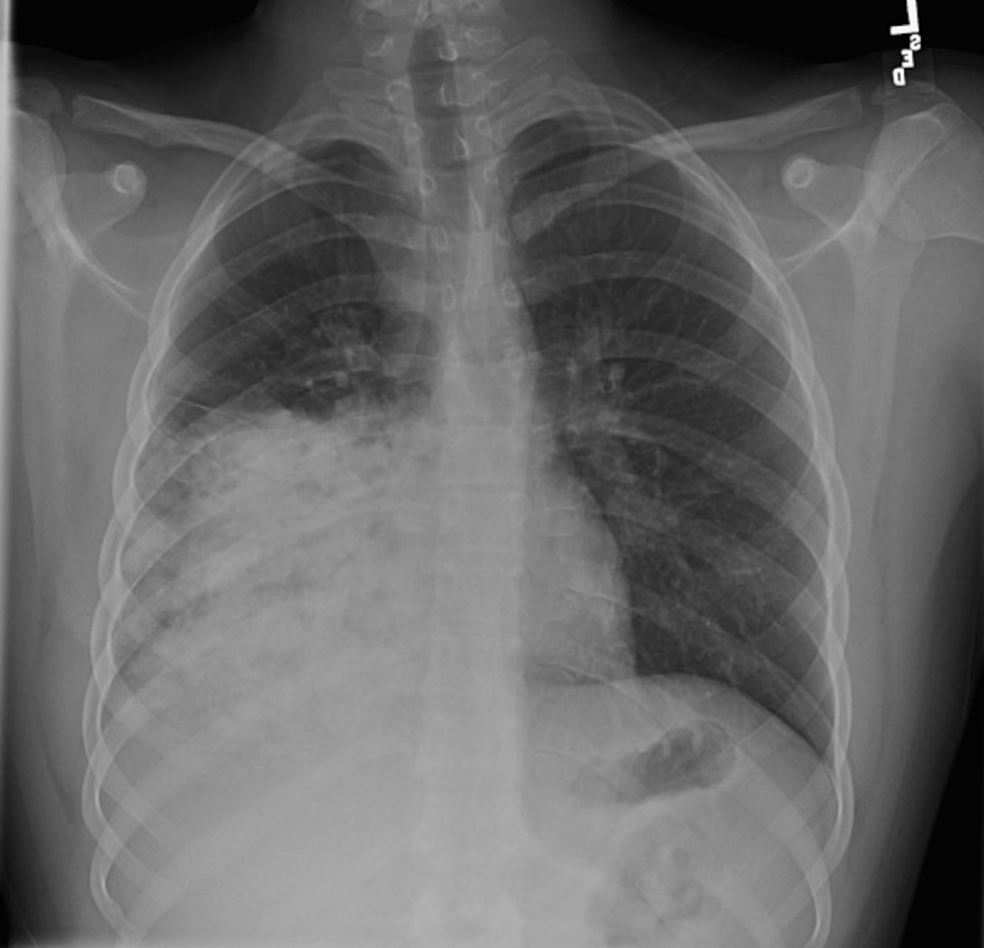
Chest X-rays on admission There was dense heterogeneous confluent opacity throughout the right middle and right lower lobe partially obscuring the right cardiac border. Trace right pleural fluid was observed.
